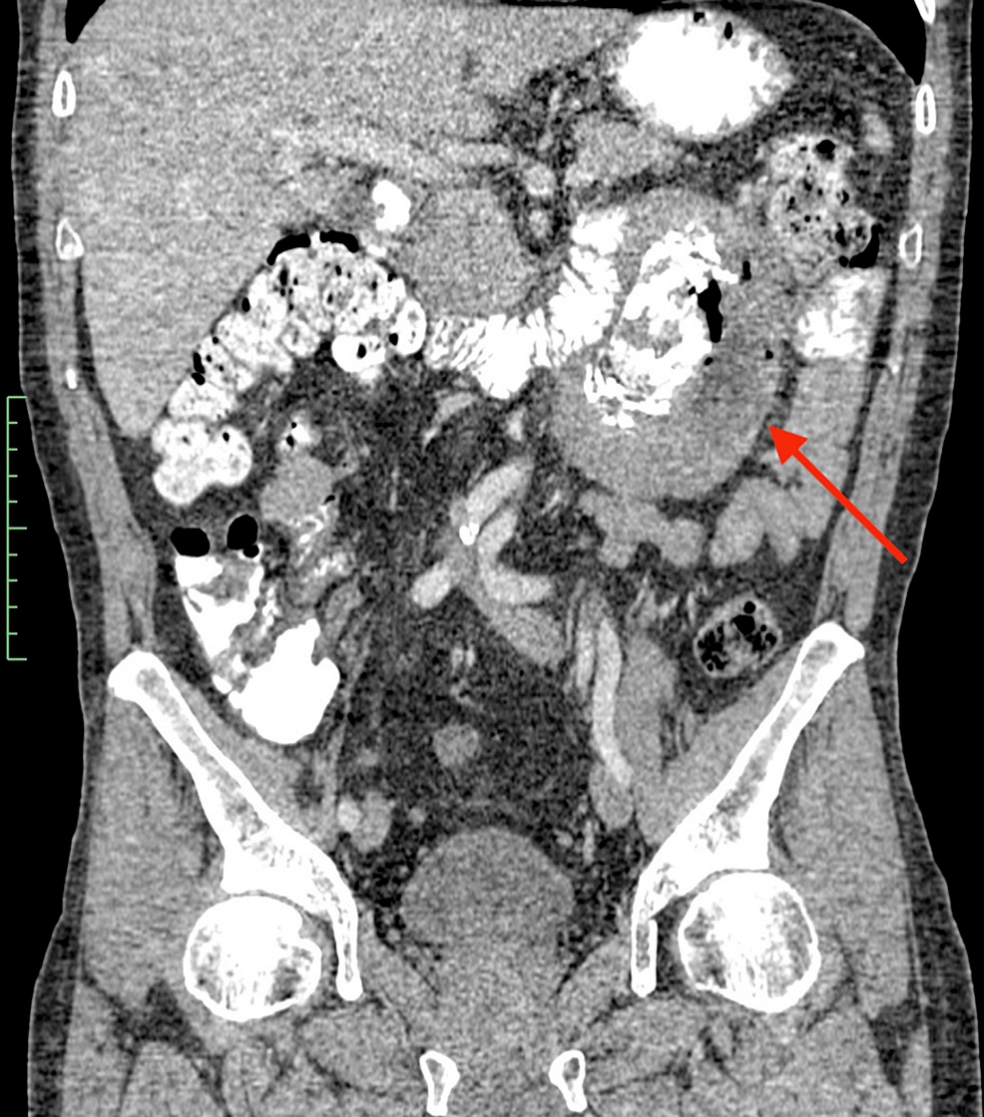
Coronal CT appearance of the retroperitoneal mass Central rings of calcification, with foci of air, are noted, probably due to direct infiltration of adjacent bowel loops CT: computed tomography
